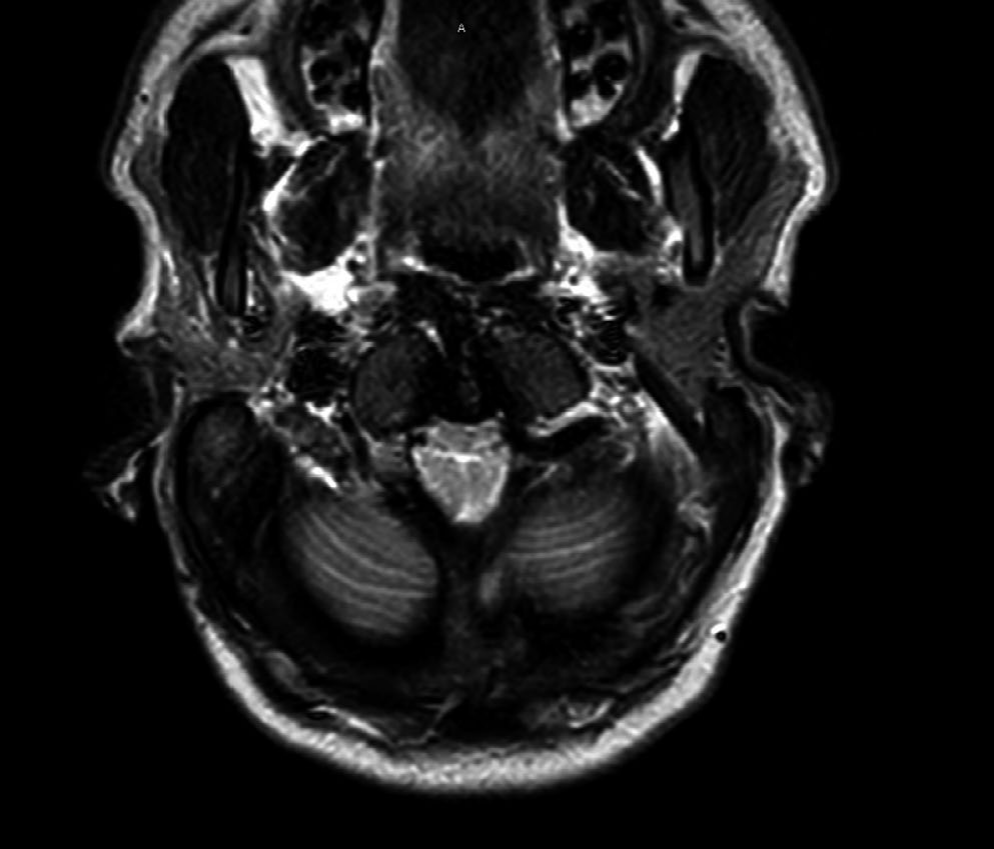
Axial C‐spine T2‐weighted magnetic resonance image of the upper cervical spinal cord at the level of C1‐2
